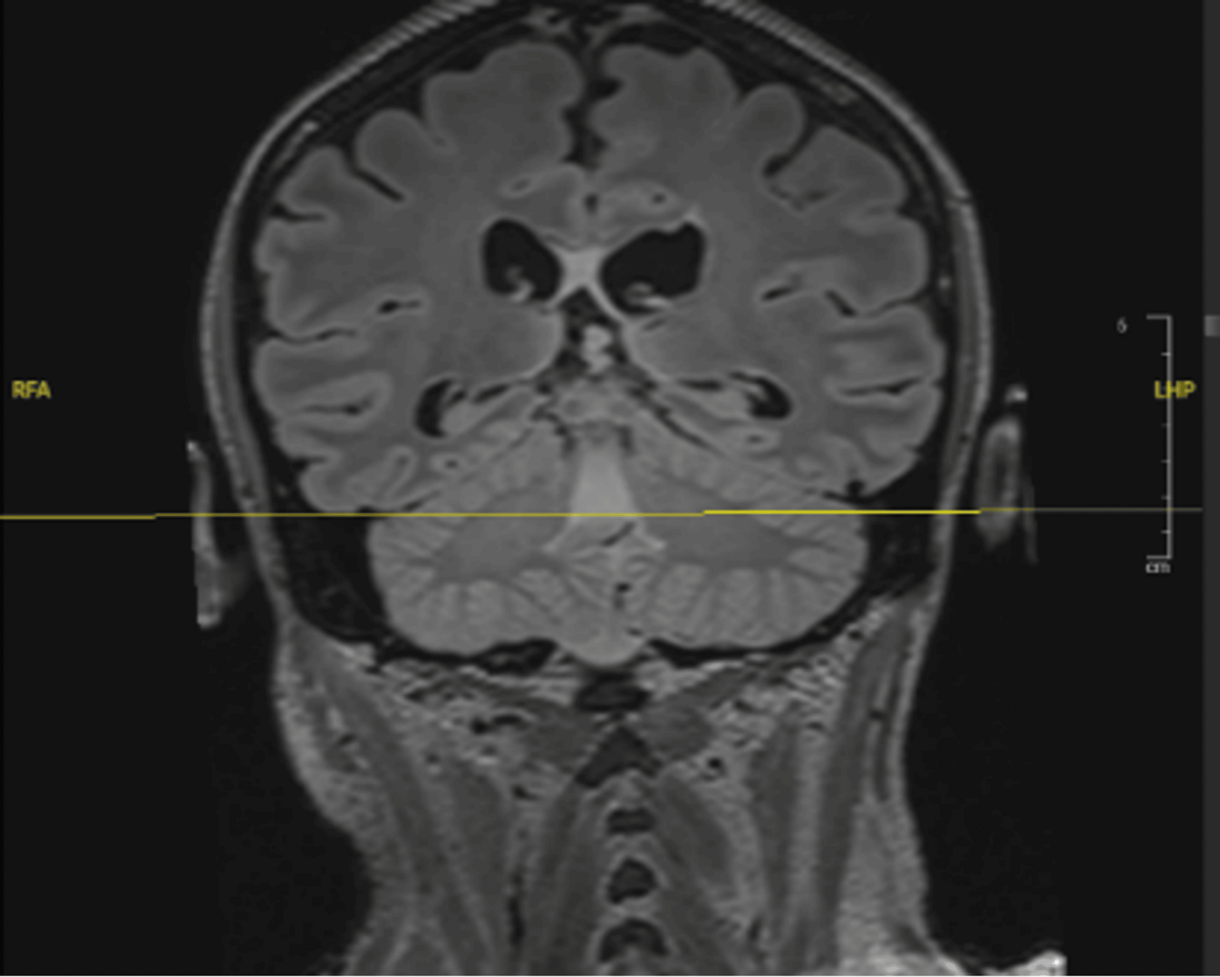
The coronal plane of the MRI revealed ventriculitis with fluid level in the fourth ventricle.
